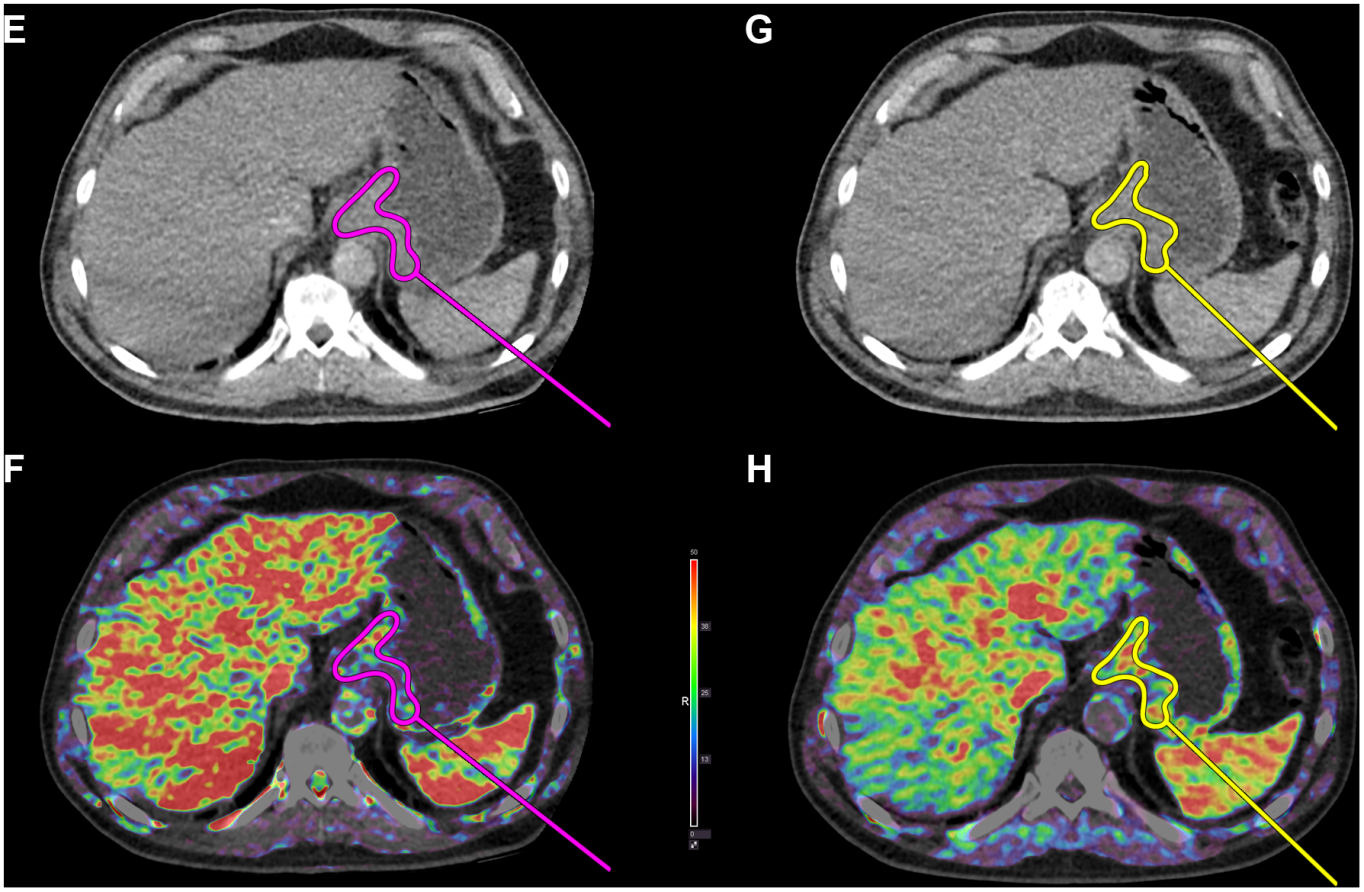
CT Perfusion images of a clinical nonresponder. Permeability k^trans^ in a clinical nonresponder. An increase in k^trans^ is observed. E+F) Before chemotherapy (k^trans^ = 13.7 mL⋅min^−1^⋅100 g^−1^) and G+H) After first series of chemotherapy (k^trans^ = 21.0 mL⋅min^−1^⋅100 g^−1^). Perfusion measures are averages from the entire tumor volume.
